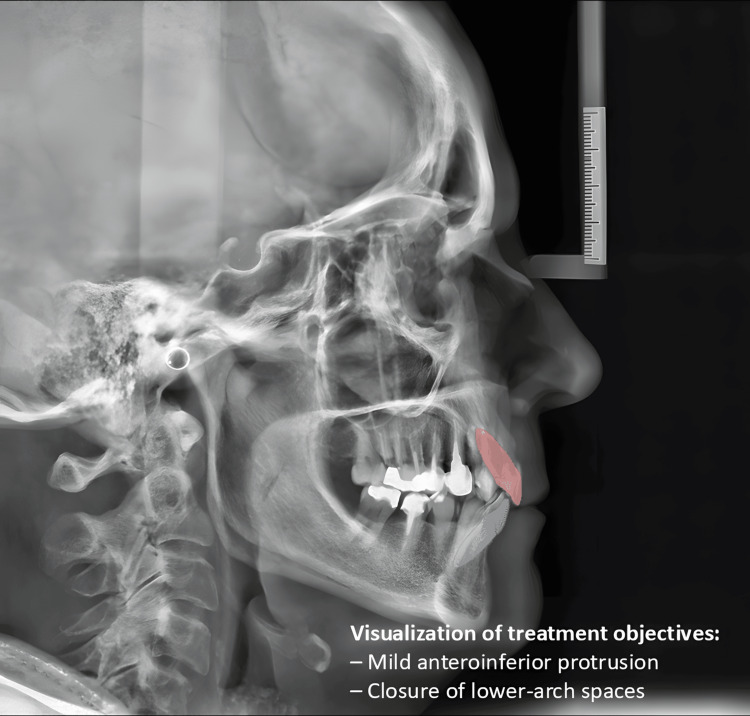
Initial lateral cephalometric radiograph with visual treatment objective superimposed, showing planned anteroposterior and vertical tooth movements
